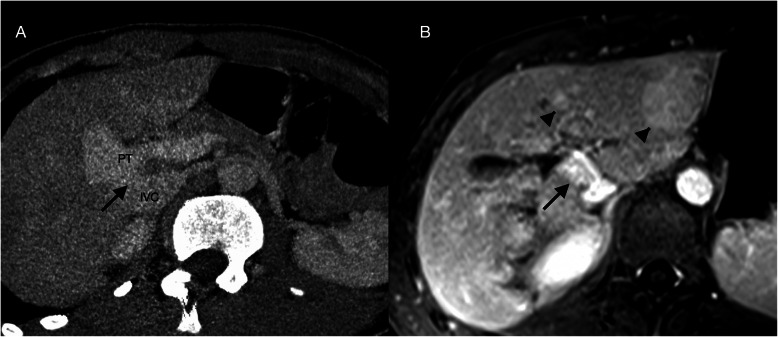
Type II congenital extrahepatic portosystemic shunt. A) CT mutiplanar reconstruction. A wide and short length shunt (black arrow) is seen connecting the inferior vena cava (IVC) and the portal trunk (PT). B) Axial equilibrium phase T1-weighted MR image with gadobutrol shows two slightly hyperintense nodules. These were also hyperintense on arterial and portal phases, compatible with the diagnosis of regenerative nodules (arrow heads)
